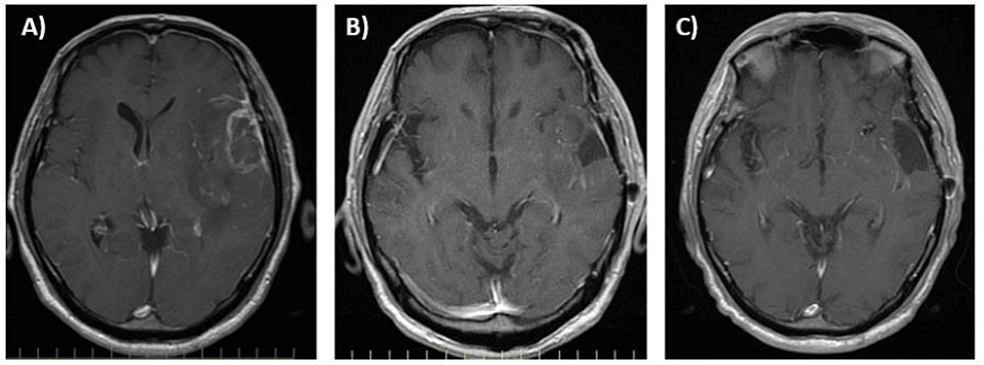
MRI brain findings (Case 2) (A) Preoperative MRI with contrast shows ring-shaped contrast-enhanced lesion in the left temporal lobe; (B) Two months after the start of maintenance therapy, no contrast lesions noted; (C) Four months after the start of maintenance, no contrast lesions noted.
